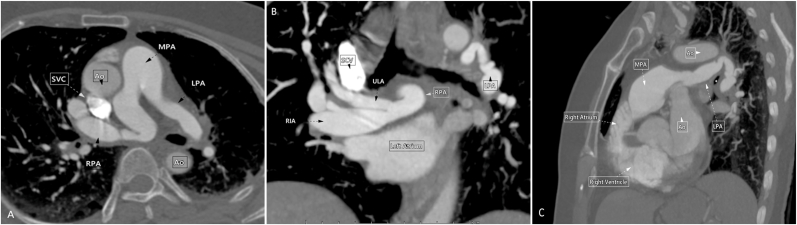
CT Angiography Revealing Pulmonary Artery Tortuosity and Segmental Loop Formation Multiplanar CT angiographic reconstruction. (A) Enlarged main pulmonary artery (MPA) bifurcating into tortuous right pulmonary artery (RPA) and left pulmonary artery (LPA). (B) The RPA exhibits marked looping prior to bifurcation into the upper lobar artery (ULA) and right interlobar artery (RIA), with caliber variation suggesting functional narrowing. (C) The LPA demonstrates looping over the left superior lobar bronchus (∗), causing mild extrinsic compression. Ao: Aorta; SVC: Superior Cava vein.
